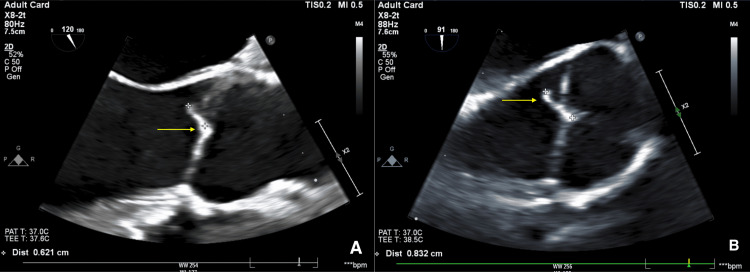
Transesophageal echocardiography showing moderate-sized mobile vegetation on the non‐coronary cusp of the aortic valve seen in two views (yellow arrows), measuring 8 mm in length (plane B).
